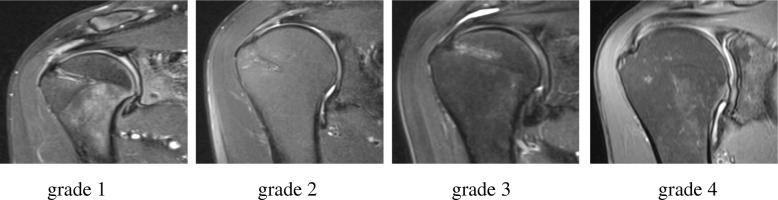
Grading of the resorption of the anchors. Grading system as described in Table 1. Coronal MRI-slices, fast spin-echo T2-weighted images with fat saturation Fat-Sat (TR/TE, 2300–4600/30–50 ms; slice thickness, 3 mm; slice gap, 0.3 mm)
